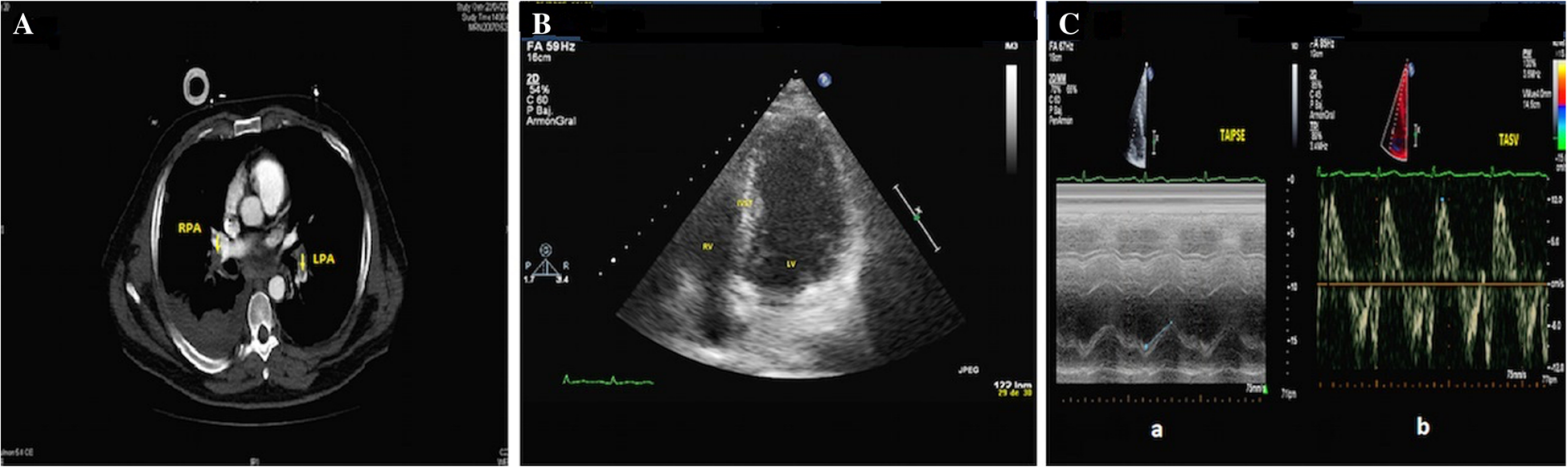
a Axial CT slice showing multiple repletion defects in both the pulmonary artery and bilateral lobar arteries, along with peripheral pulmonary consolidations with hypodense zones compatible with areas of hypoperfusion. b Four-chamber apical view of TTE showing the right ventricle with normal diameter and contractility after PE treatment. c Normal (a) TAPSE and (b) TASV as an expression of functionality of the normal right ventricle after PE treatment. *CT* Computed tomography, *TTE* Transthoracic echocardiography, *PE* Pulmonary thromboembolism, *TAPSE* Tricuspid annular plane systolic excursion, *TASV* Tricuspid annular systolic velocity
